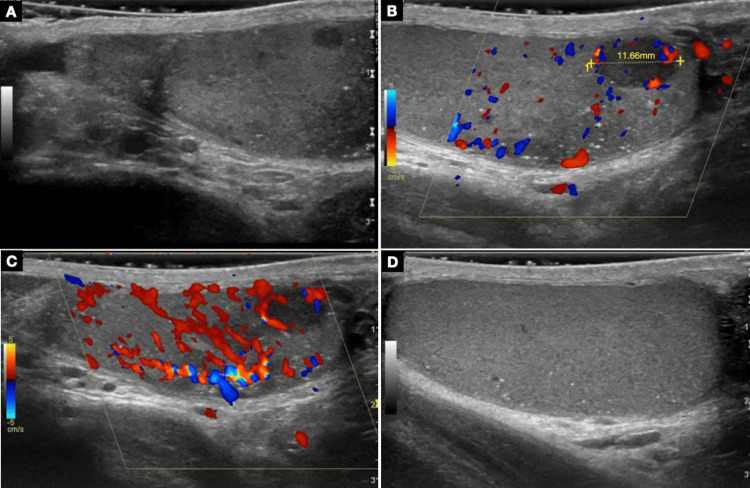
Scrotal ultrasonography of the left testis showing a markedly heterogeneous structure, with hypo-echoic areas, (A) microlithiasis, and (B), (C) an 11.7 mm well-circumscribed, hypervascular nodule. (D) Normal right testis.
